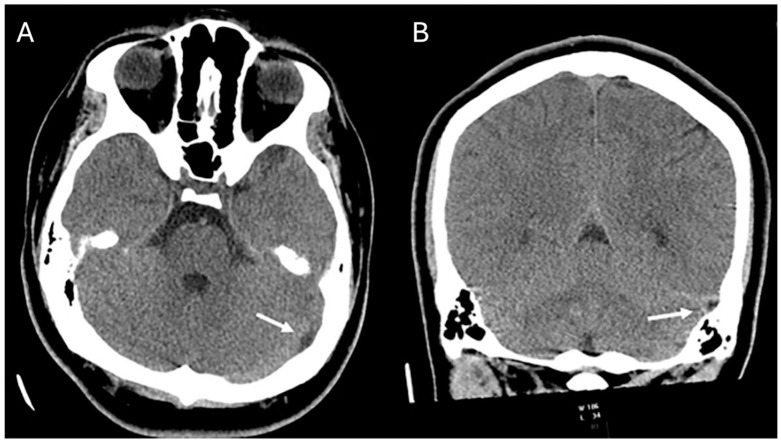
Axial (A) and coronal (B) CT images demonstrate a hypodense area along the course of the left sigmoid and transverse sinus (arrows) compatible with a CSF filled Pacchionian granulation extending into the lumen of the affected dural sinuses.
